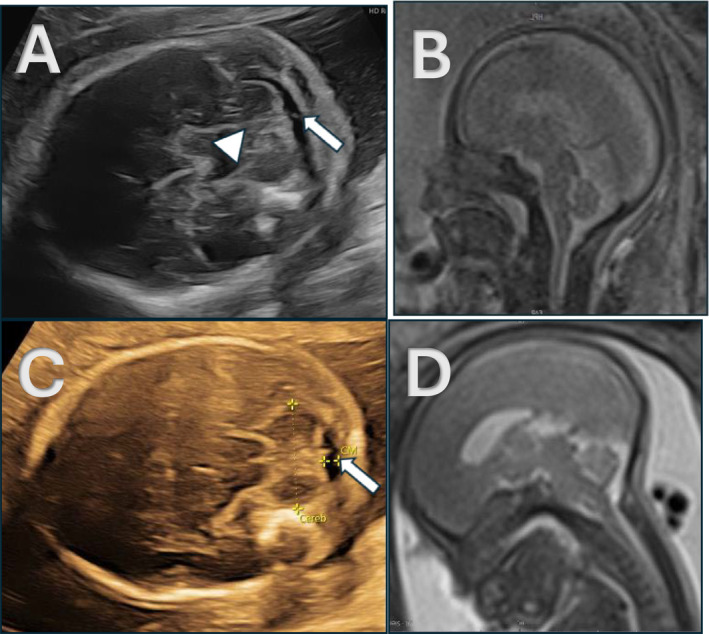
(A–D) Prenatal cranial US and MRI of case numbers 1 and 5. (A, B) Prenatal US and T2‐weighted MRI of fetal brain of case number 1 at 22.9 weeks GA, showing a normal‐shaped cerebellum and a patent fourth ventricle (arrowhead) and cisterna magna (arrow). No HBH is visible on fetal MRI. (C, D) Prenatal US and T2‐weighted MRI of fetal brain of case number 5 at 20.6 weeks GA, showing a normal‐shaped cerebellum and a patent cisterna magna (arrow). No HBH is visible on fetal MRI.
